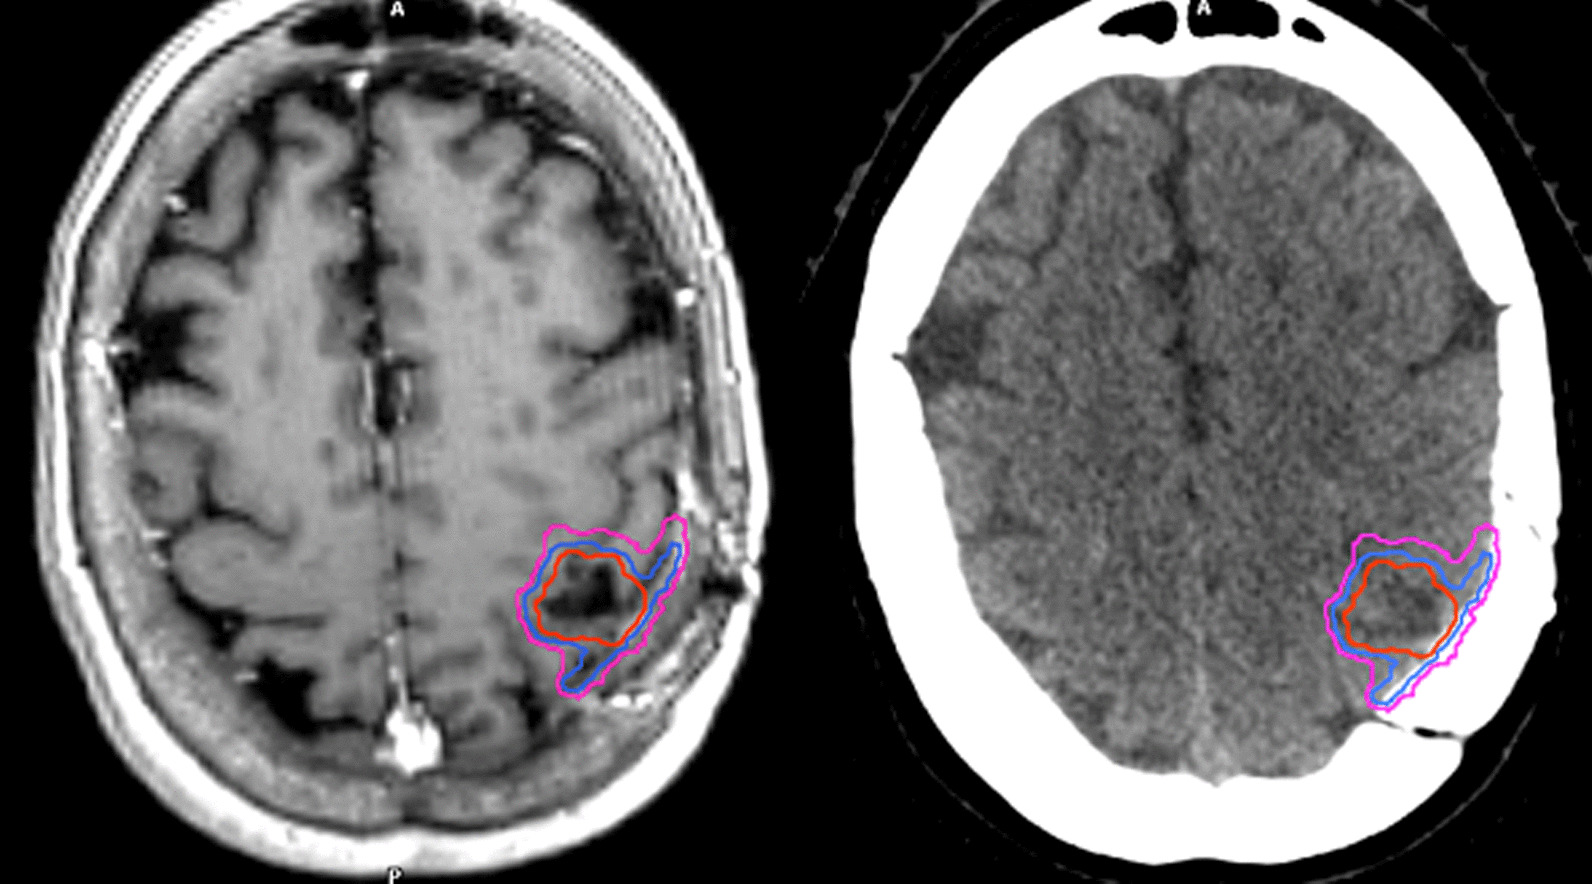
An overview of target volumes for postoperative resection cavity is presented on post-contrast T1-weighted MRI sequences and CT scans. The gross tumor volume (GTV) is presented in red, the clinical target volume (CTV) in blue and the planning tumor volume (PTV) in pink. For this case, CTV was created by 1-mm expansion of the GTV, extended by 5 mm along the bone flap beyond the initial region of preoperative tumor contact. Note that an extension by 10 mm along the meningeal margin for brain metastases with preoperative dural contact [59] or the inclusion of the entirety of the craniotomy site [71] has been suggested by some authors
